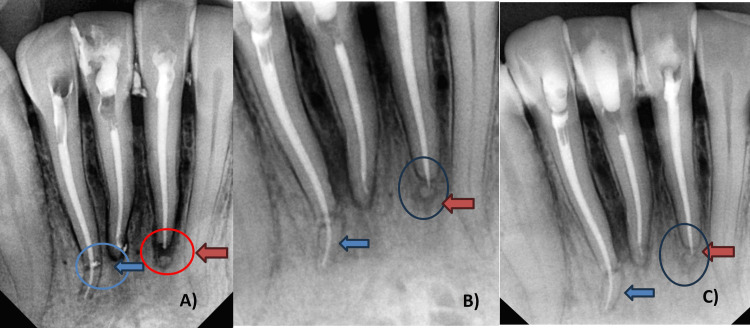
Follow-up radiographs A) Immediate post-obturation radiograph (blue circle and arrow show unified segments in 42, and red circle and arrow show obturation in 31). B) After six months (blue arrow in 42 and red arrow with circle in 32 show positive healing). C) After one year (red arrow and circle in 31 and blue arrow in 42 show positive healing)
